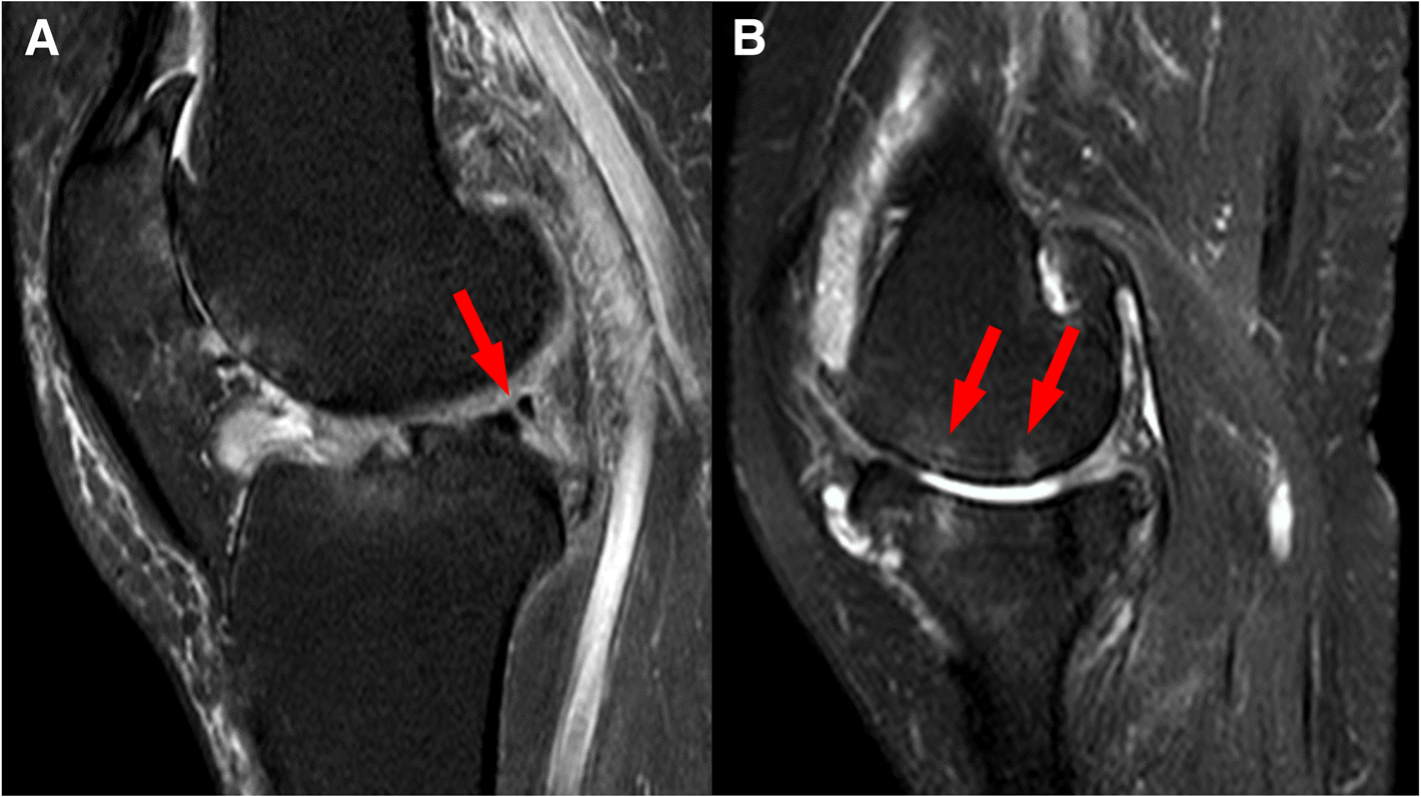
The MRI results of a 49-year-old female patient. a Red arrow indicates the longitudinal tear of posterior horn of medial meniscus. b Red arrows show the cystic degeneration and subchondral bone osteonecrosis. The cartilage damage can also be observed
